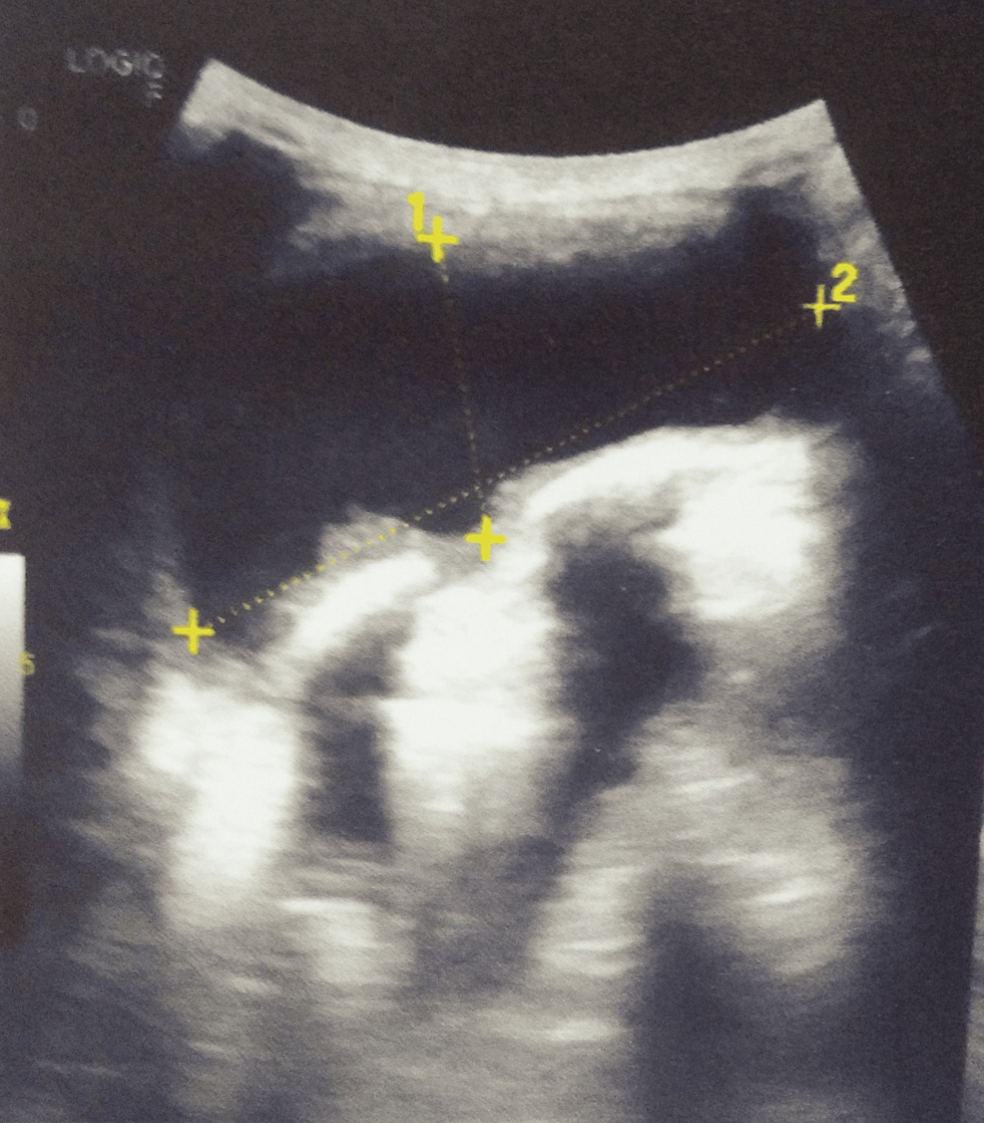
Ultrasonography image of the chest Ultrasonography of the chest showing loculated collection (approximately 52 cc).
